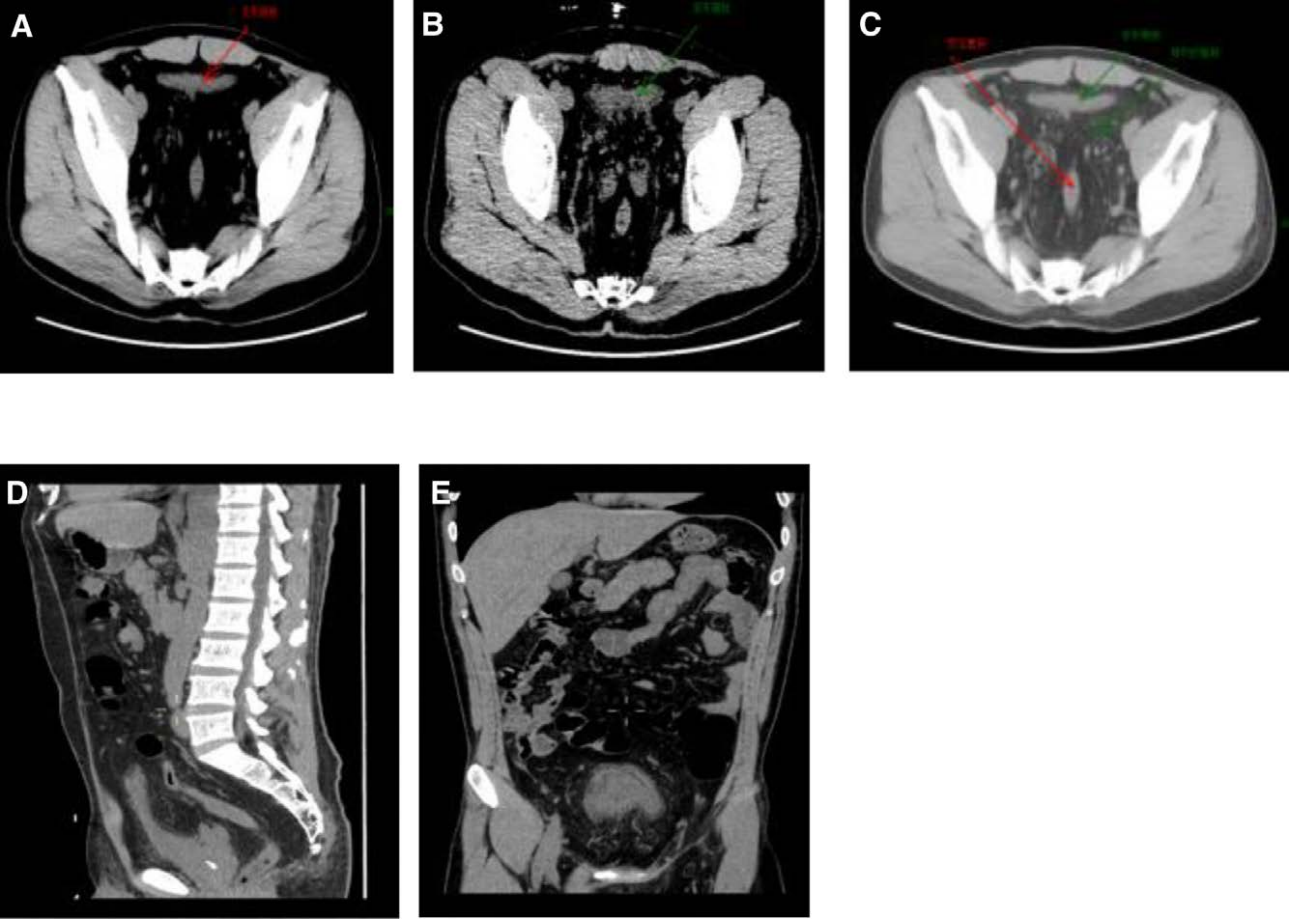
(A–E) Full abdomen + pelvic CT: (A–C) Horizontal CT scans show diffuse low-density fat accumulation around the bladder and rectum (pelvic transparency sign), with compression and deformation of the bladder and rectum, and poor bladder filling. The bladder wall is thickened and rough, and there are tortuous pelvic veins. (D, E) Sagittal and coronal CT scans show elevation and deformation of the bladder base in the sagittal view.
